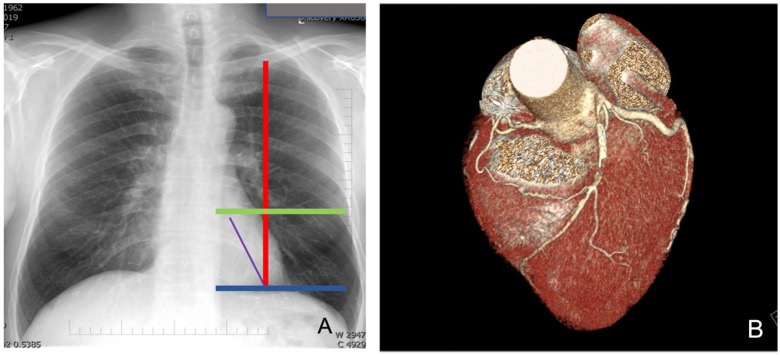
Preoperative chest X-ray (A) and CT-scan with 3D reconstruction (B).
